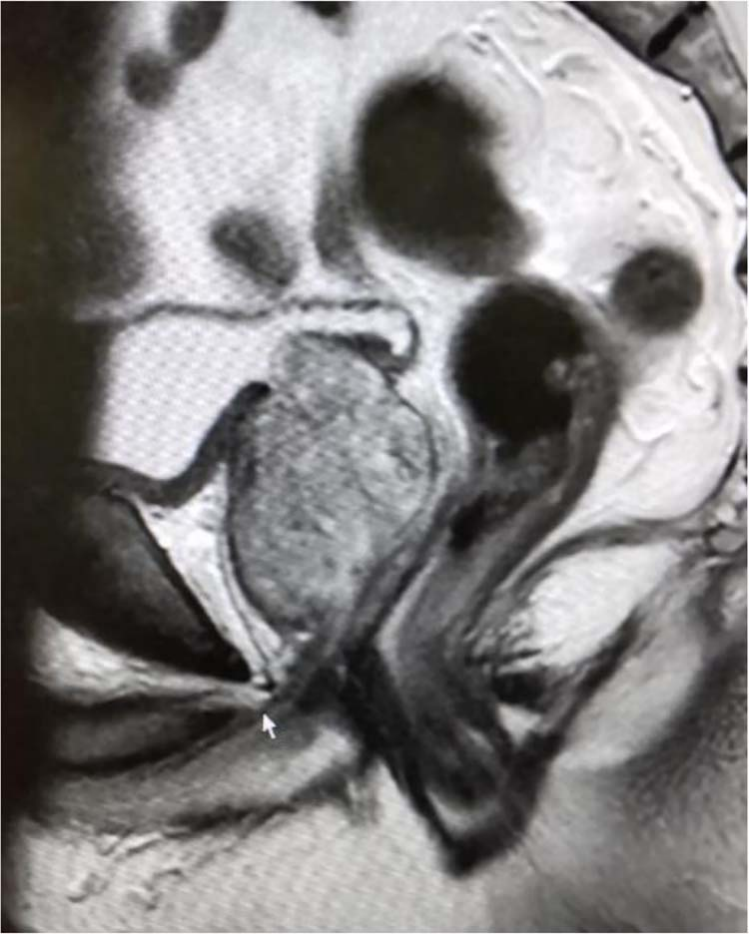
Magnetic resonance imaging before surgery showing BPH with middle lobe hypertrophy.
